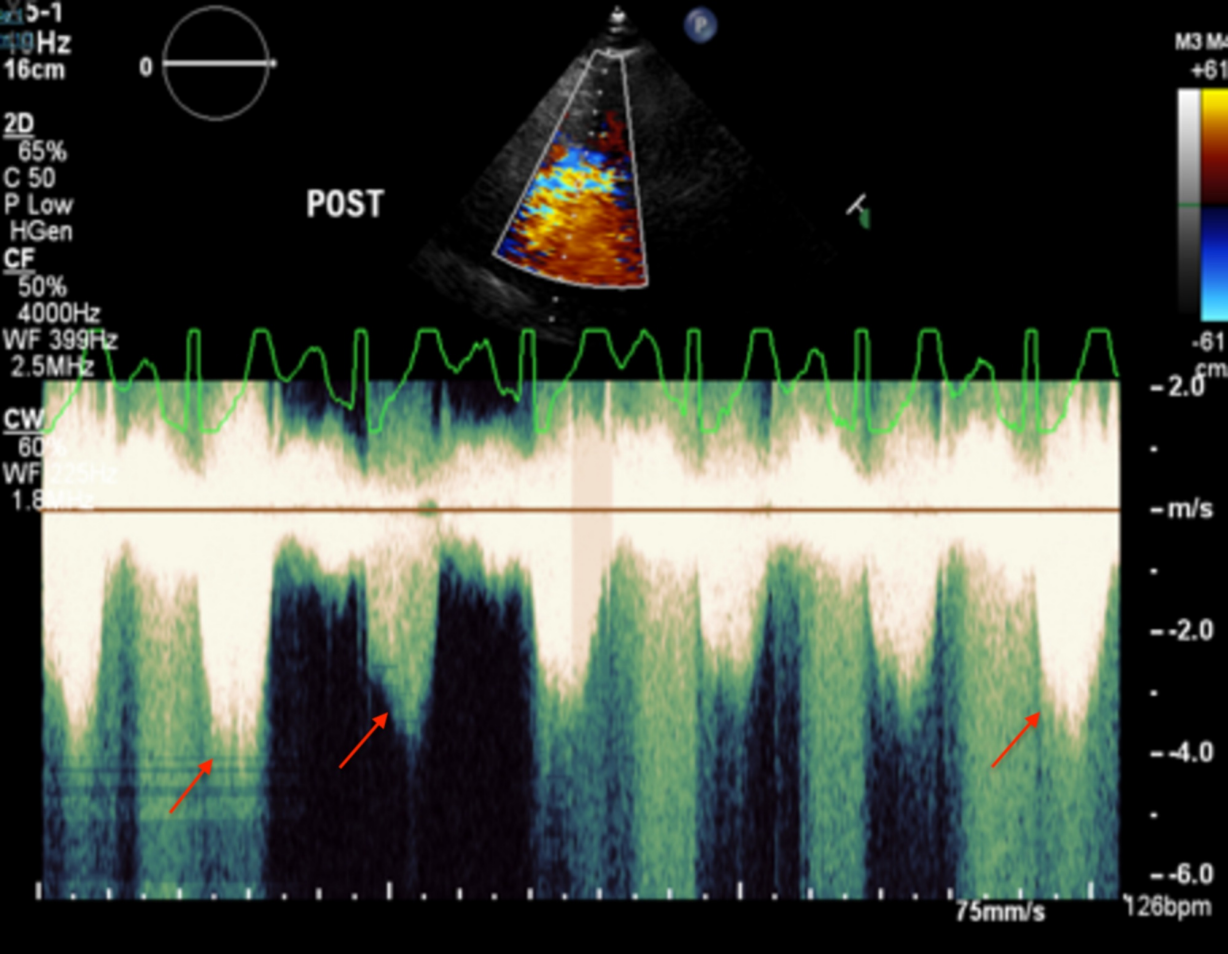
Post-exercise echocardiography showing late peaking dagger shaped transient left ventricle outflow tract obstruction (red arrows)
